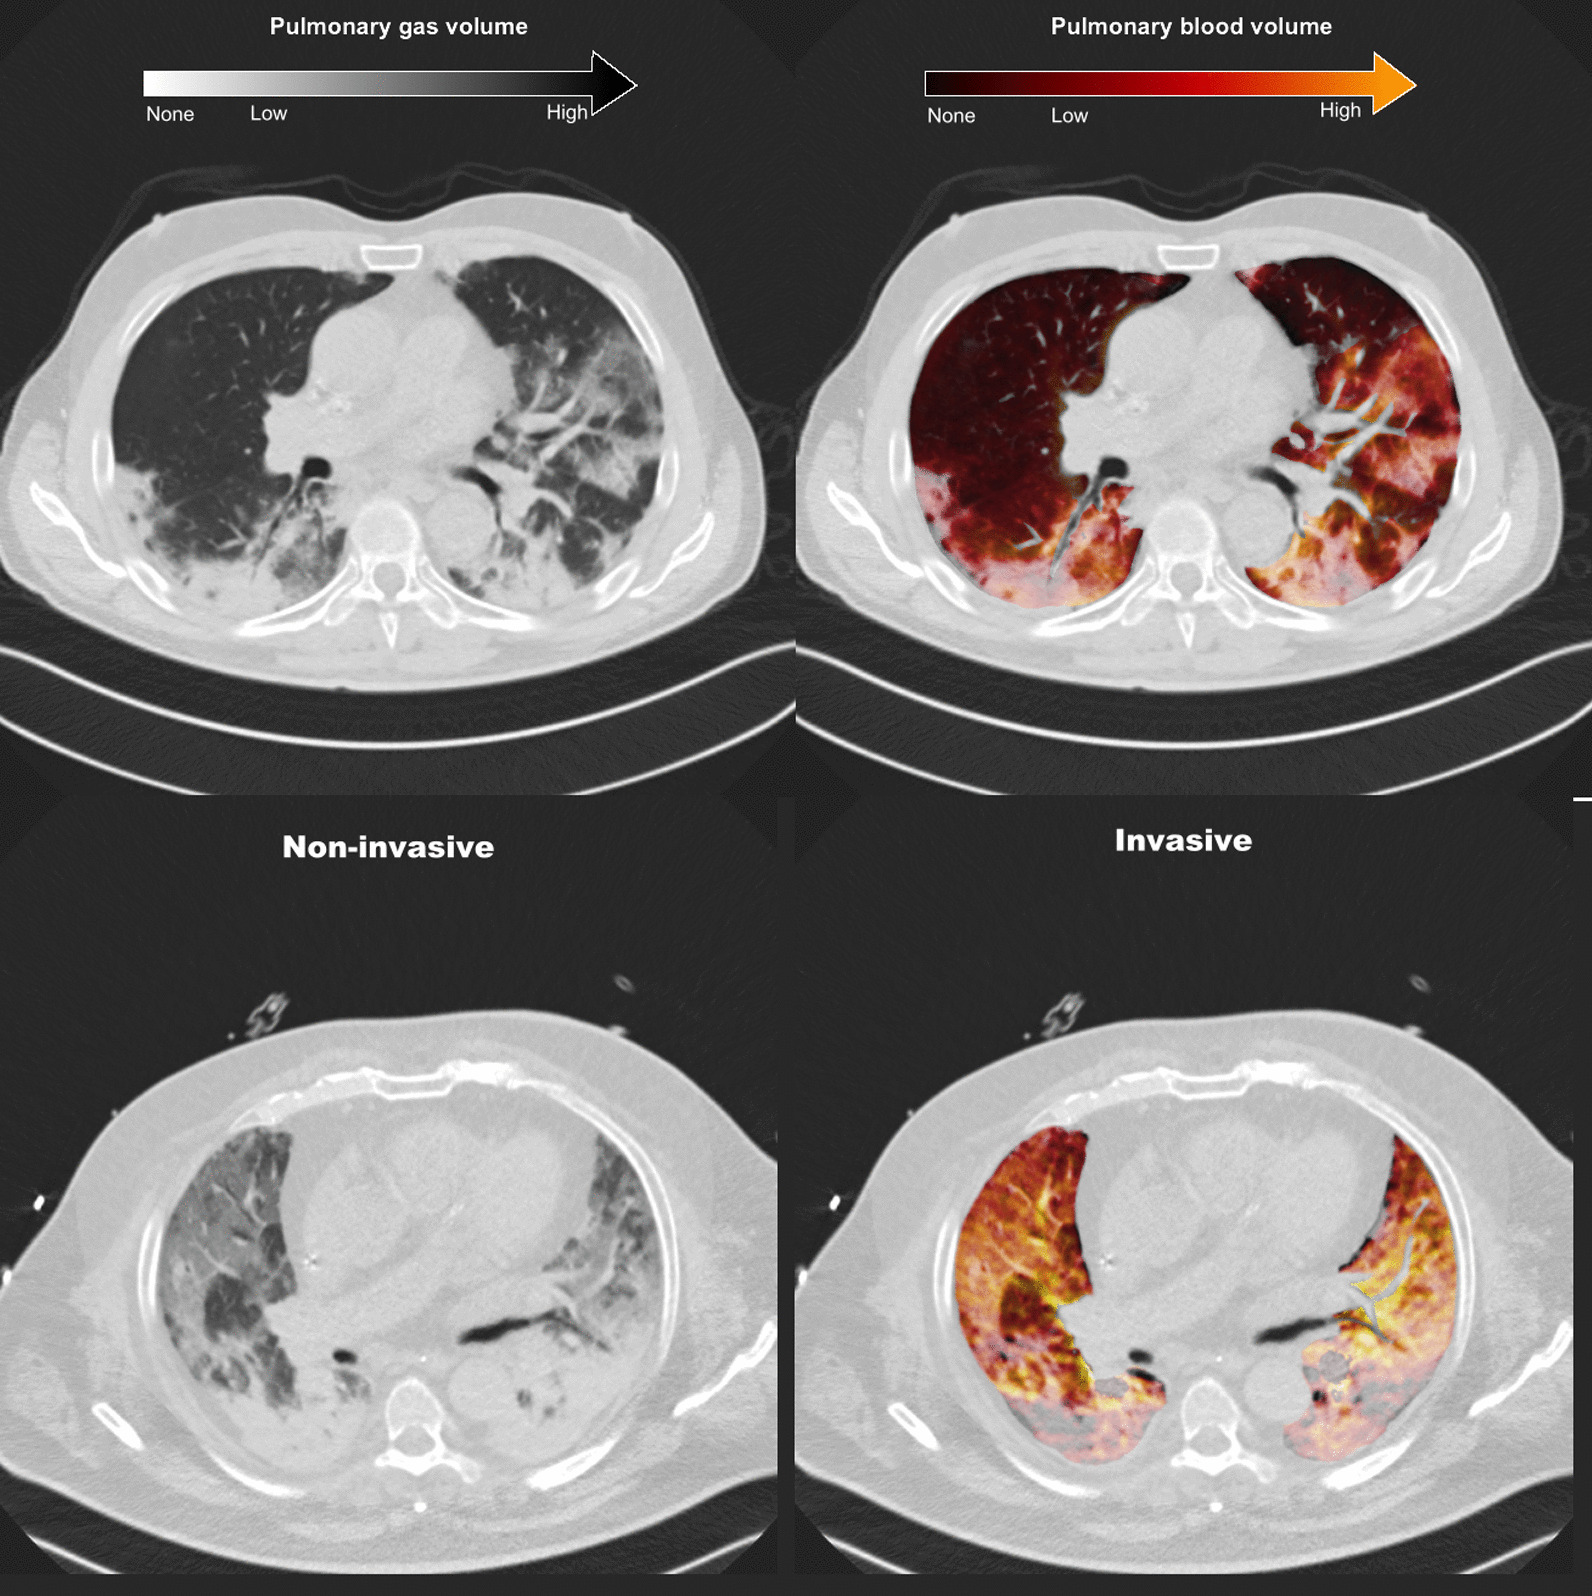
Representative DECT scans of patients receiving non-invasive (top panels) and invasive (bottom panels) respiratory support. The images on the left represent the virtual non-contrast image used for the assessment of aeration, while those on the right represent the pulmonary blood volume map superimposed onto the virtual non-contrast image. The patient in non-invasive ventilation shows areas of ground-glass with both high (yellow) and low (gray) pulmonary blood volume. The patient receiving invasive ventilation shows a more advanced disease characterized by diffuse ground-glass and consolidative lesions, with vast areas of lack of pulmonary blood volume (grey zones) especially in the dorsal dependent regions. *DECT* dual-energy computed tomography
